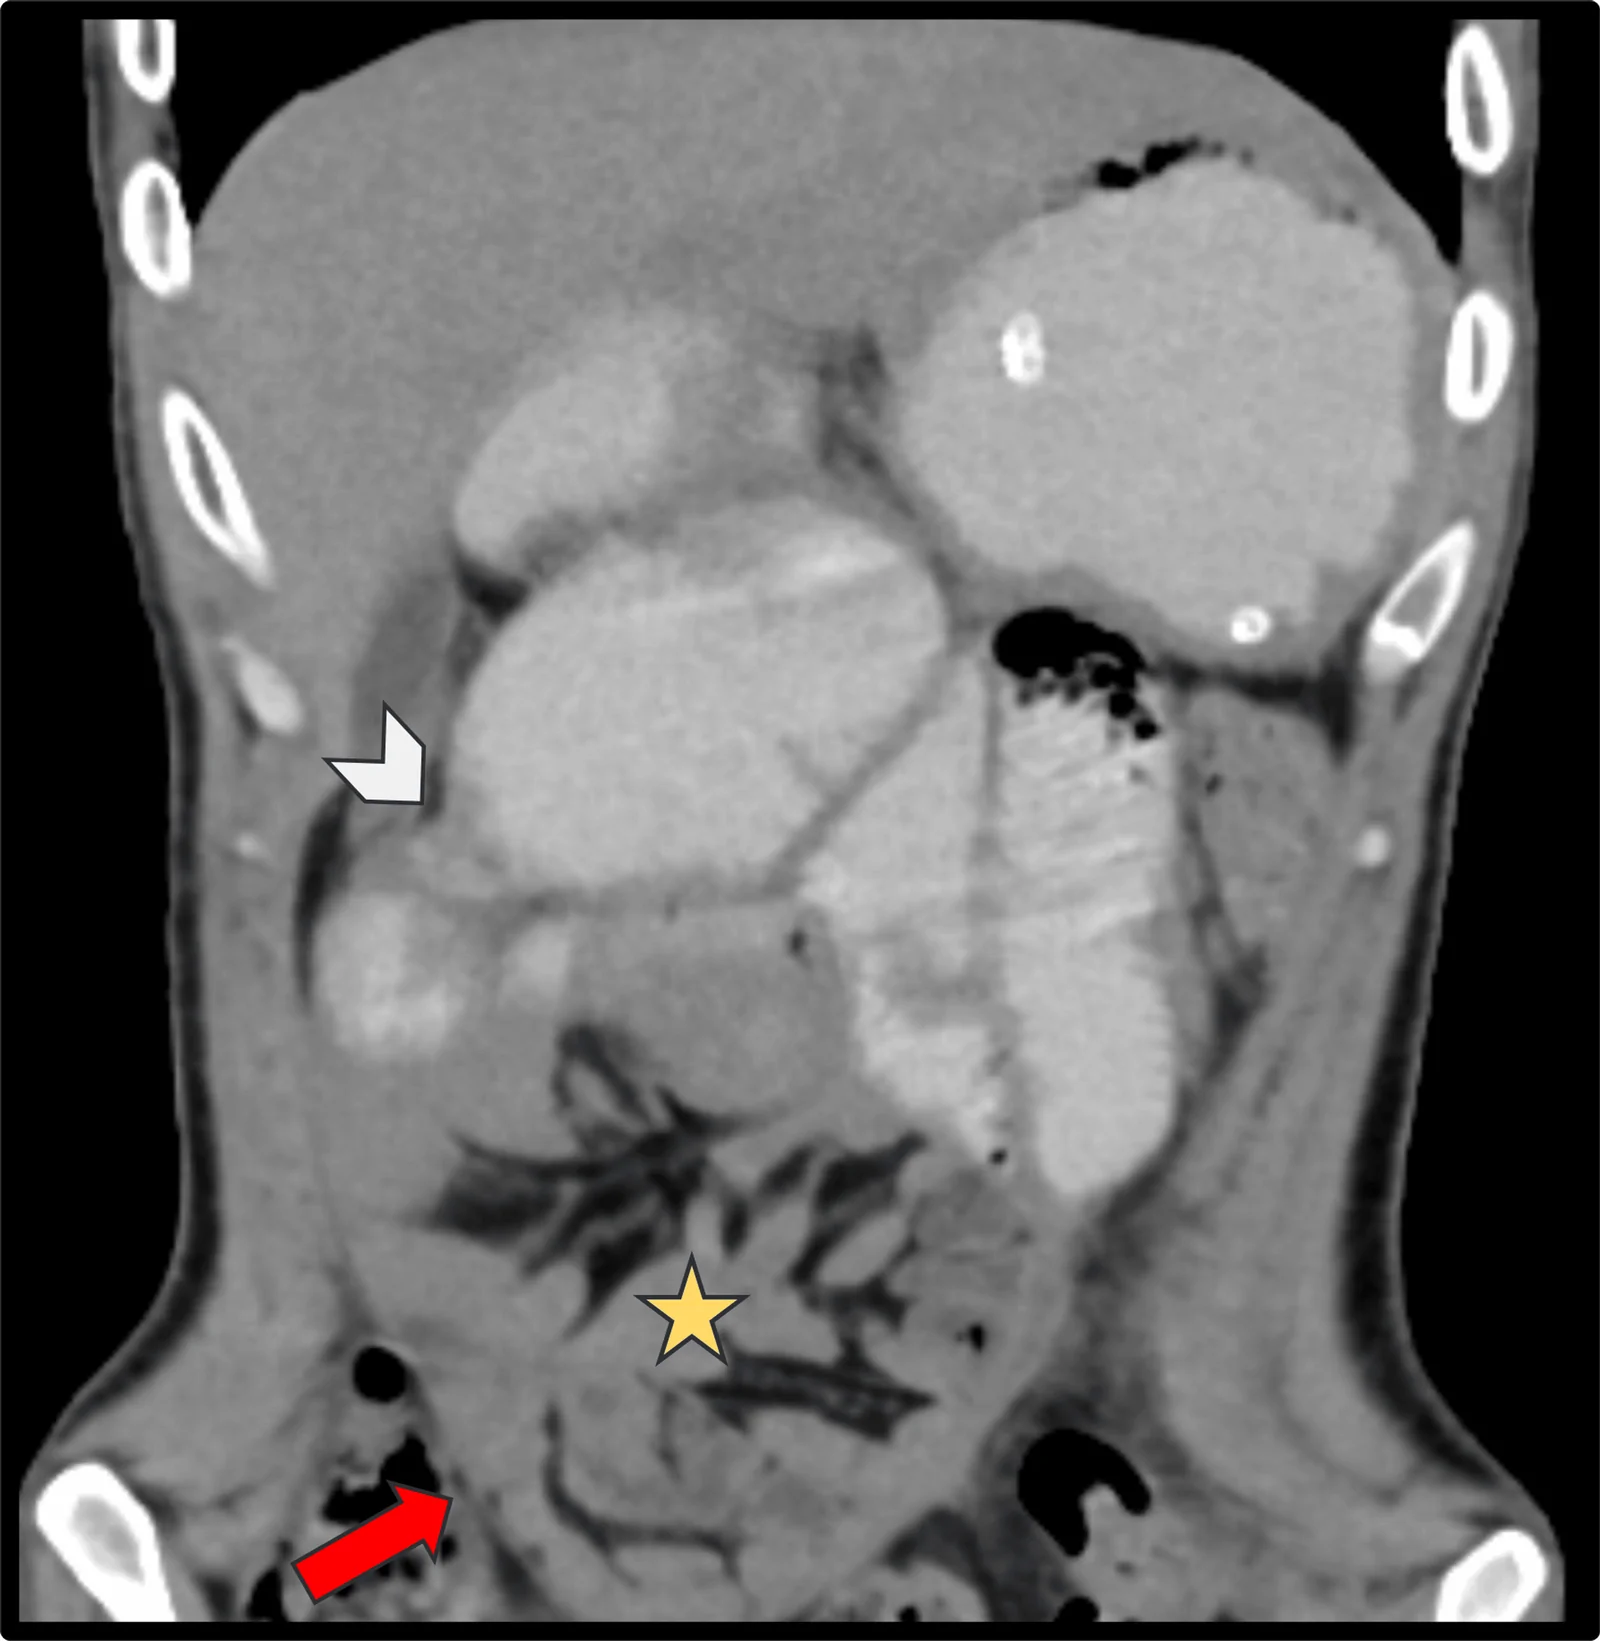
Coronal reconstructed CT image showing the transition point at the site of the transmesocolic internal hernia (white arrowhead). A separate focus of sclerosing EP is seen in the lower abdomen, with clustered small bowel loops (yellow star) enclosed by a fibro-collagenous membrane (red arrow), confirming the coexistence of two distinct pathologies EP: encapsulating peritonitis
